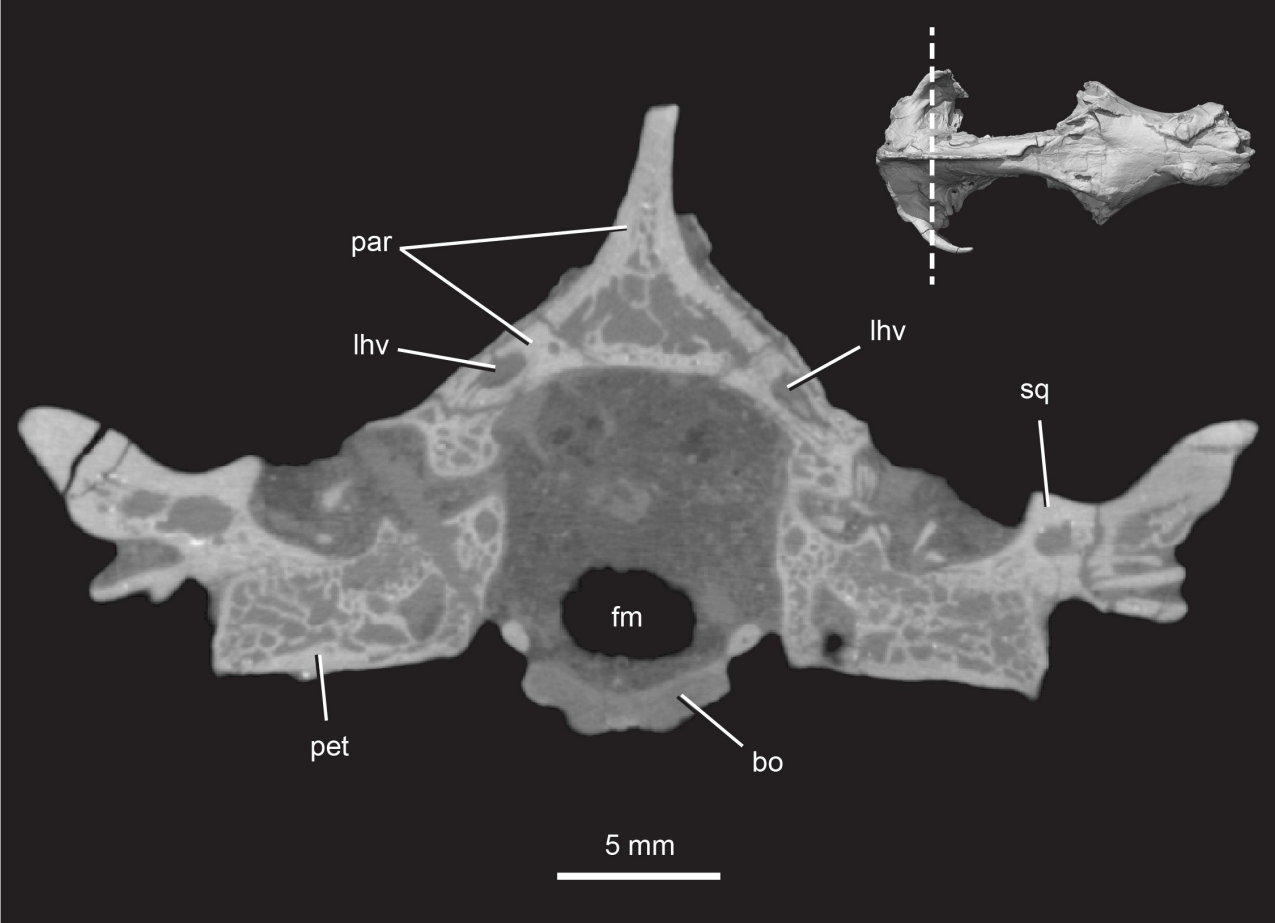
Pseudotherium argentinus*, CT cross section through the back of the skull of the skull. Note the extensive hollow spaces in the parietal, petrosal, and squamosal. Abbreviations: bo, basioccipital; fm, foramen magnum; lhv, lateral head vein; par, parietal; pet, petrosal; sq, squamosal.
